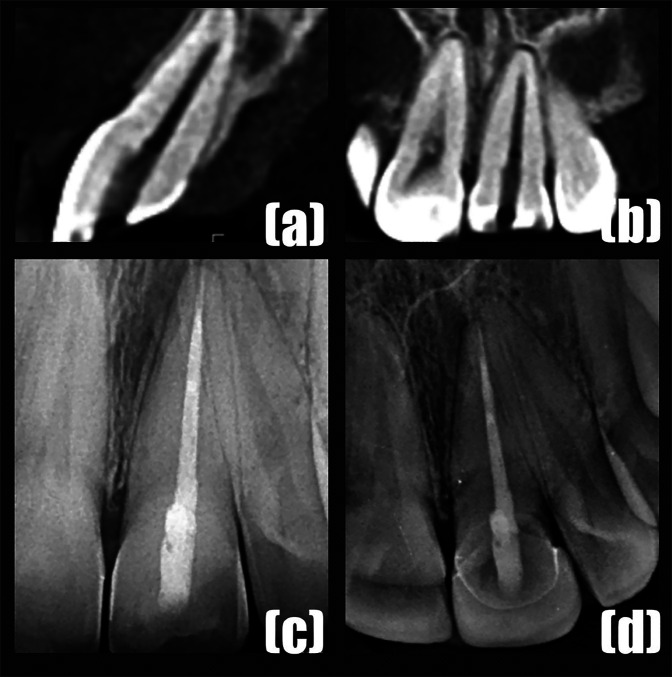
(a-b) CBCT showing the presence of apical deviation. (b) Postoperative periapical radiograph. (d) Follow-up radiograph Two year after the completion of the root canal treatment.
